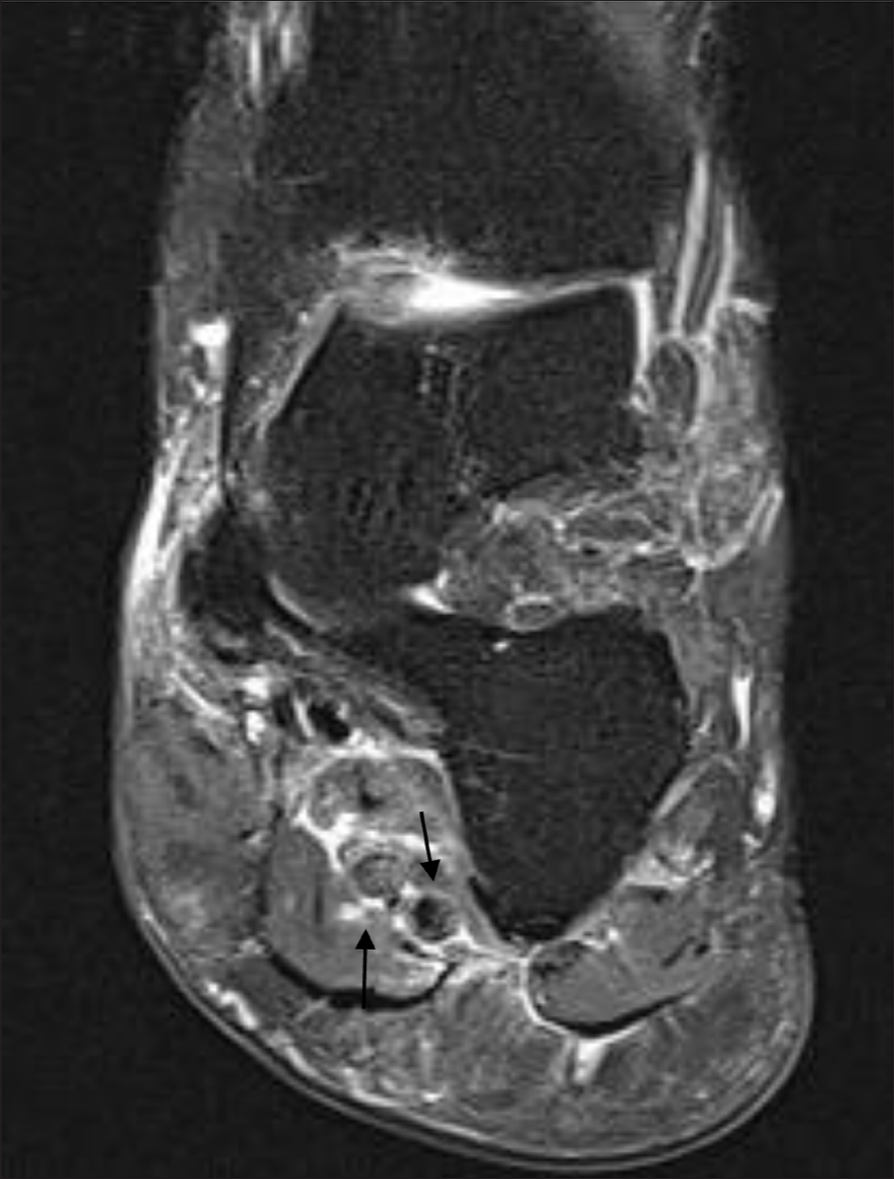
Subsequent color Doppler ultrasound examination showed absence of venous flow signal with intravenous hypo-echogenic clot formation within dilated noncompressible plantar veins (*Figure 3*, A coronal B sagittal, black arrows).
